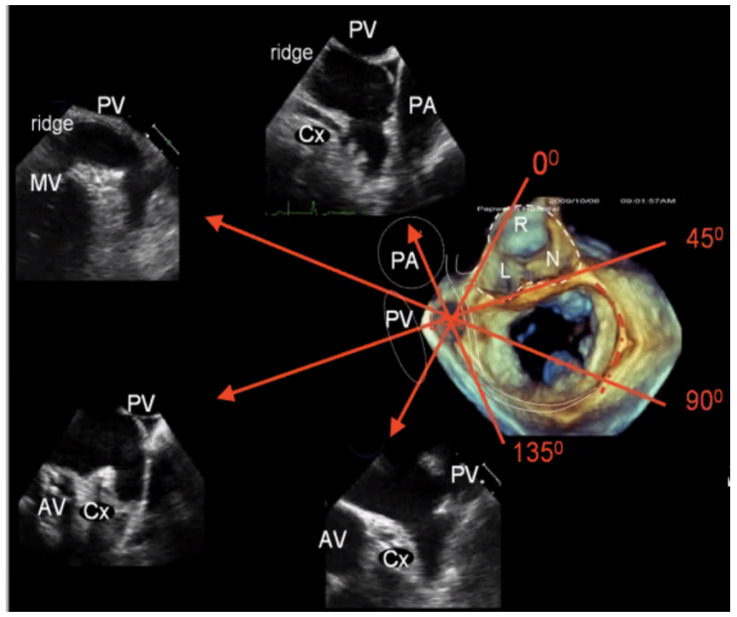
Conventional 2D transoesophageal echo view (0, 45, 90, and 135°) of the left atrial appendage and surrounding structures. AV: Aortic valve; MV: Mitral valve; PA: Pulmonary artery; PV: Pulmonary vein; Cx: Left circumflex artery.
